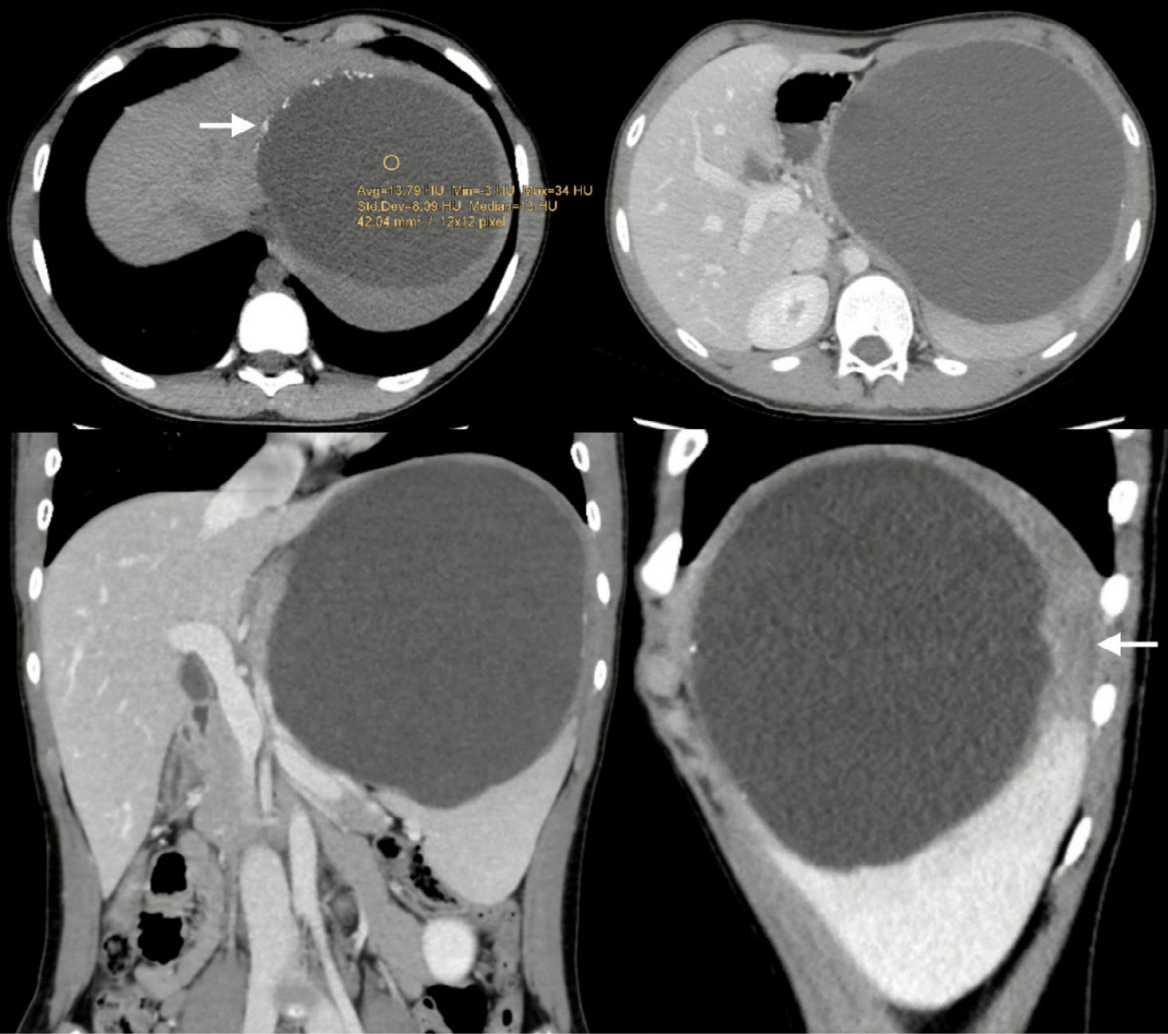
Contrast enhanced CT scan of the upper abdomen showing enlarged spleen with well‐defined hypodense lesion (HU: 14) at the upper pole of the spleen, measuring 13.5 × 14.5 × 16 cm with mass effect upon the adjacent structures. It shows peripheral discontinuous wall calcification (a, arrow); however, no soft tissue or fat component. The upper pole of the spleen is showing hypoenhancement (d, arrow) likely due to edema or infarction.
